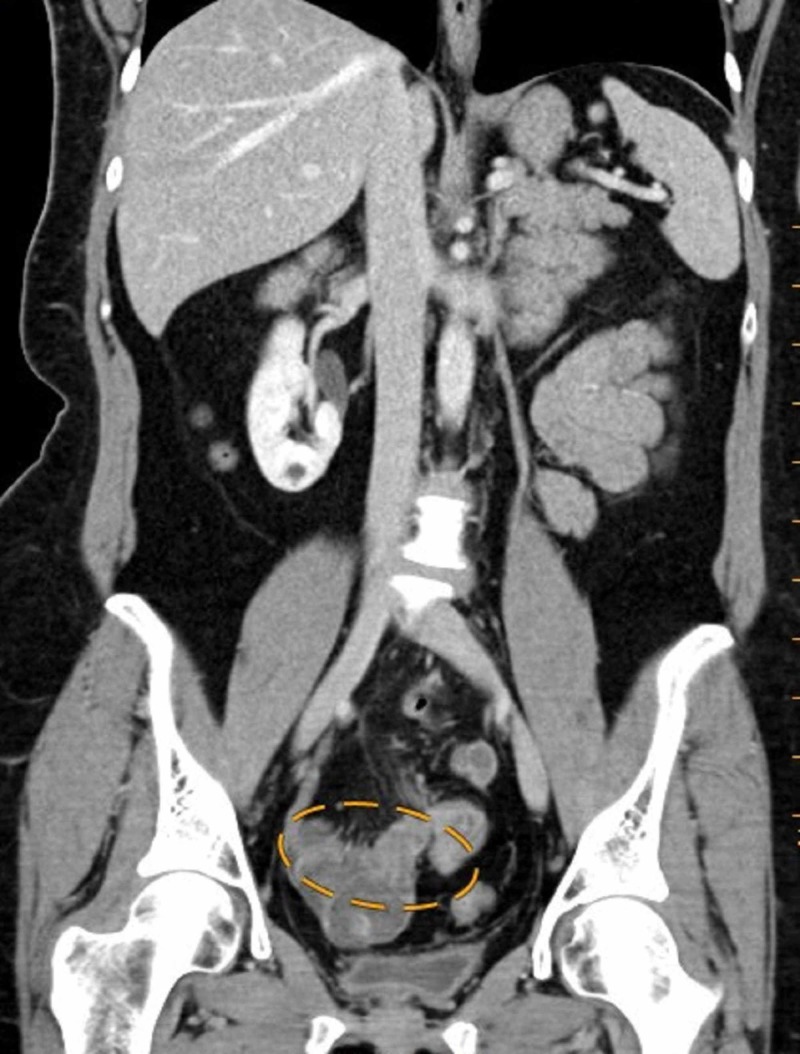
Coronal computed tomography of the abdomen and pelvis revealing a thick, irregular appendix periappendiceal stranding concerning for acute appendicitis (yellow oval ring).
